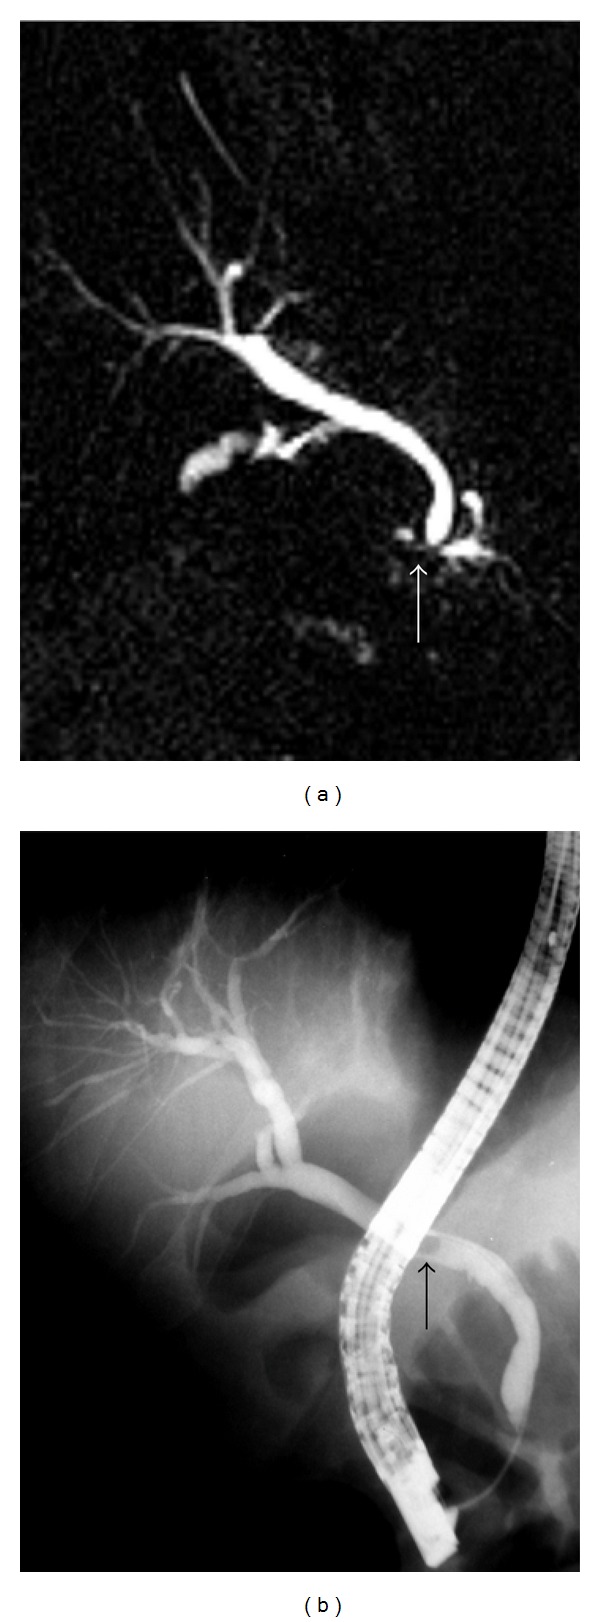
MRCP image demonstrates (a) a calculus (white arrow) located in the distal common bile that does not cause bile duct dilatation. This calculus was not detected during the MRI examination and received a false negative diagnosis. (b) ERCP image demonstrates the calculus (black arrow). This calculus was extracted during the procedure.
